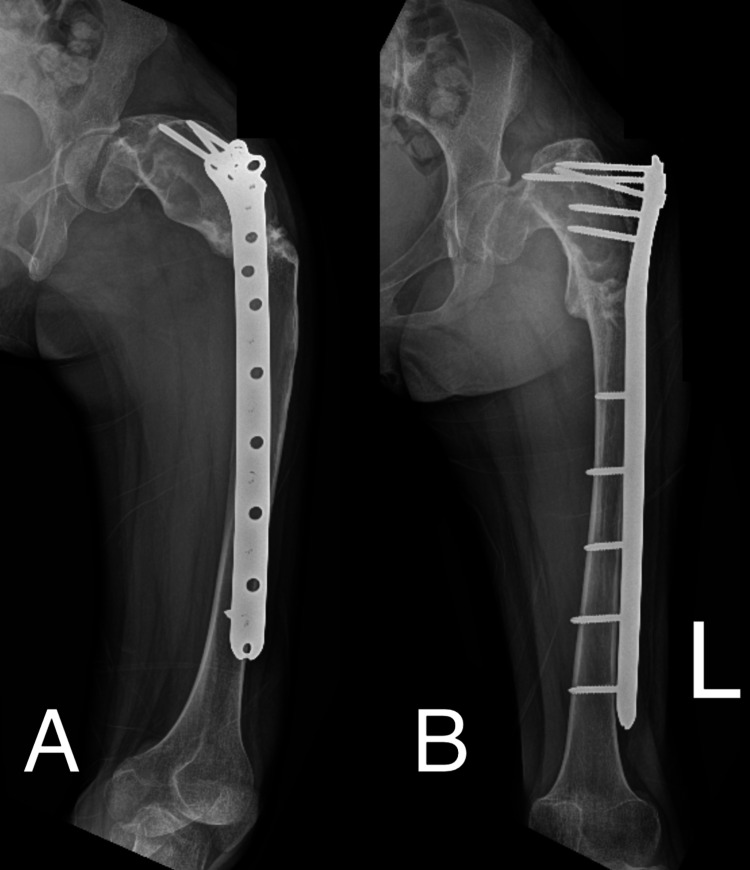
Radiograph showing lateral (A) and AP (B) view of the left lower limb during the first-year postop follow-up. AP: Anteroposterior
